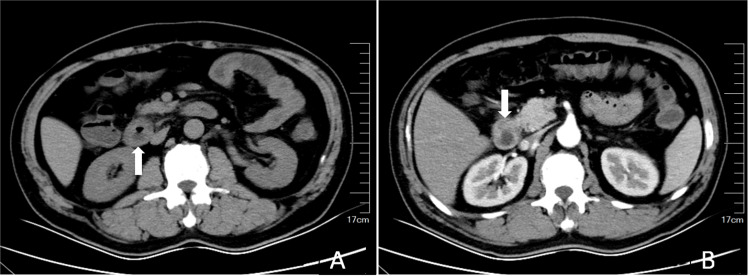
Contrast-enhanced CT scan of the upper abdomen: plain phase (A) and arterial phase (B). CT scan showed signs of thickening of the descending part of the duodenal wall (white arrow). CT: computed tomography
